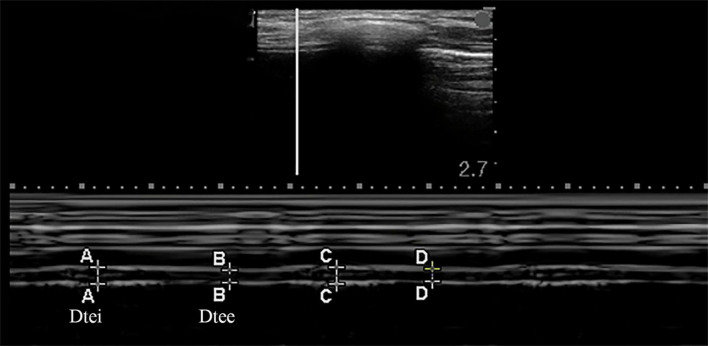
Measurements of the diaphragm thickness by the ultrasound in M-mode. Dtee, diaphragm thickness at the end of expiration; Dtei, diaphragm thickness at the end of inspiration.
